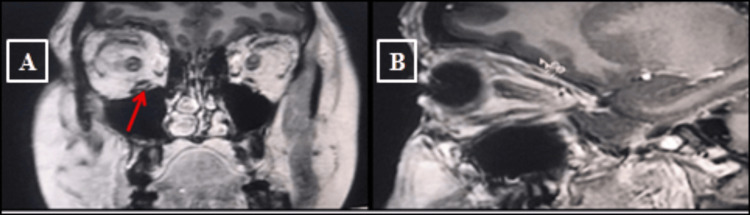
Coronal and sagittal CT scans of the orbits showing enlargement of the right inferior rectus muscle without optic nerve compression . (A) Coronal CT scan of the orbits showing enlargement of the right inferior rectus muscle (highlighted by the red arrow); (B) Sagittal CT view confirming focal hypertrophy of the same muscle. The optic nerves and globes appear normal. These findings are consistent with unilateral thyroid-associated orbitopathy (TAO) and underlying Graves’ disease.
